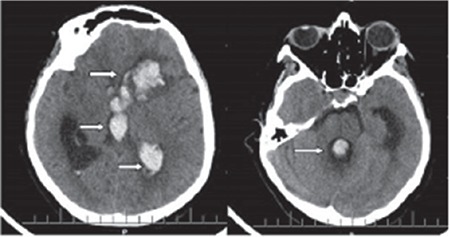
The patients preoperative axial brain computed tomography showing hemorrhage in the left lateral, third, and fourth ventricles, and parenchyma
